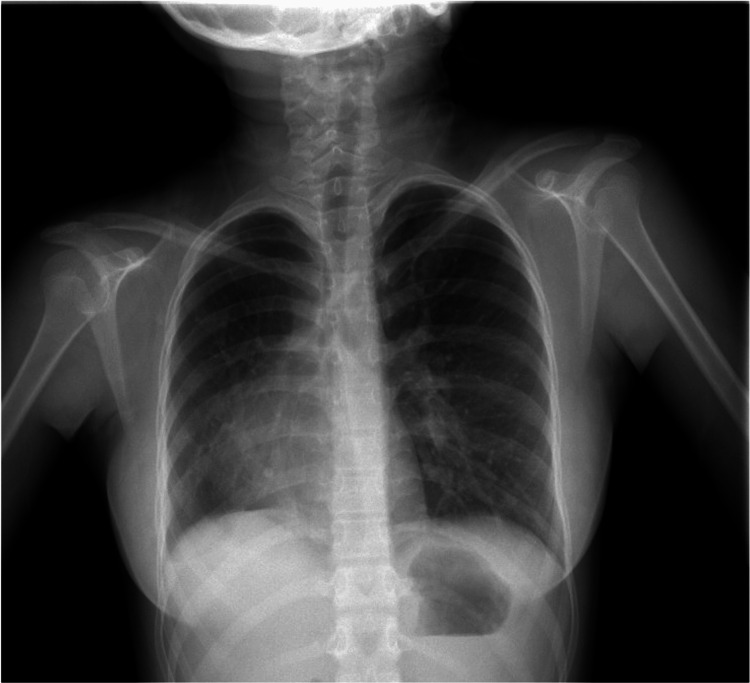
Chest X-ray posteroanterior view in scimitar syndrome. Chest X-ray taken at eight years of age shows dextroposition of the cardiac silhouette and a smaller right lung.
